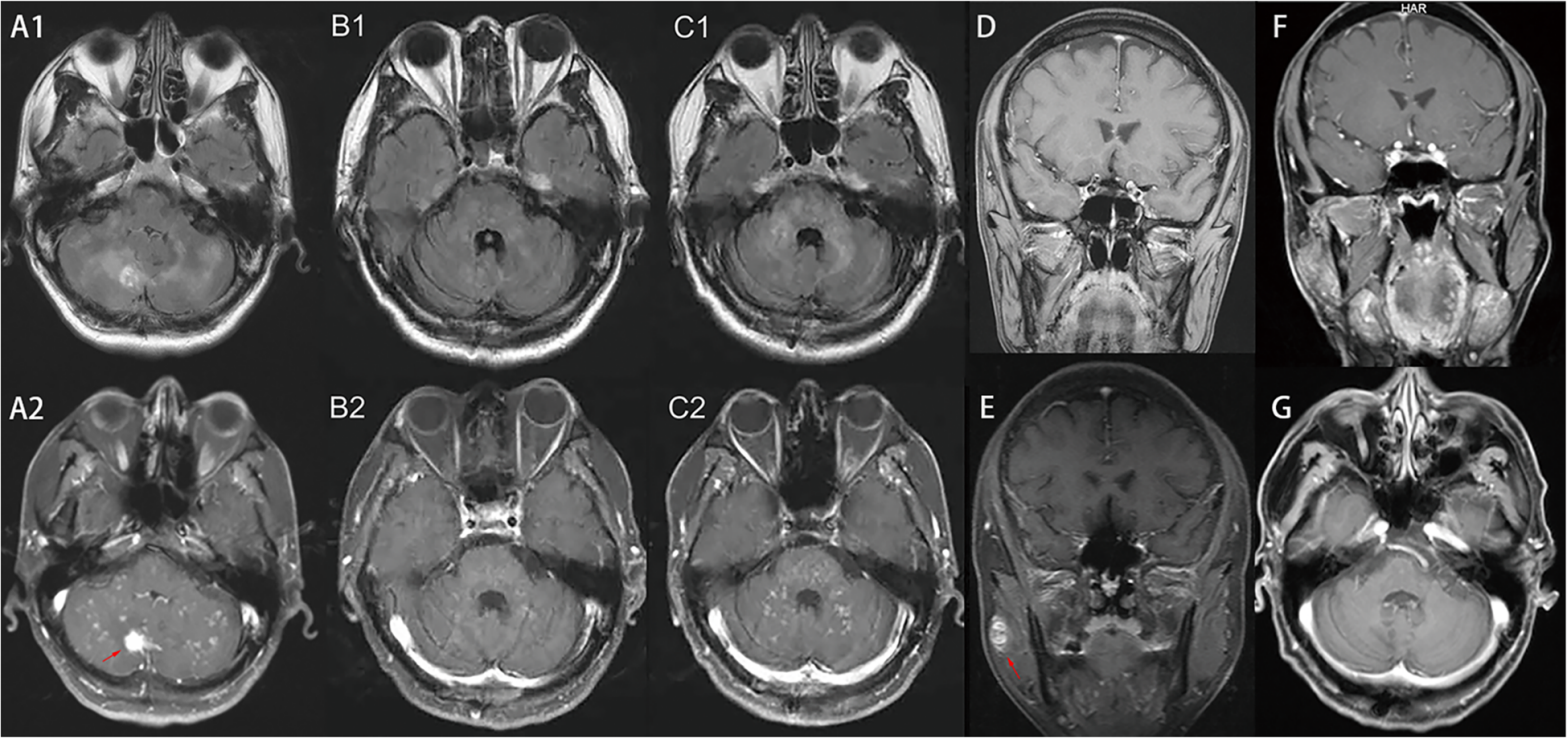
Radiological findings. Flair images (A1) and Axial contrast-enhanced T1-weighted (A2) showed punctate and curvilinear enhancing lesions “peppering” on pons and cerebellum, with a large one in 1.07 cm diameter located in the right cerebellar hemisphere (arrow). There was no parotid malignancy observed at that time (D). With pulse therapy of intravenous methylprednisolone and following oral prednisolone, subsequent MRI showed the lesions decreased both in size and amount (B1, B2). When the dosage of prednisolone was tapered to 20 mg two months later, MRI showed recurring lesions (C1, C2). An enhancing nodule of 1.8 cm diameter was found in right masseter muscle (arrow) (E). After the resection of parotid malignancy (F), with adjuvant radiotherapy, PCGE lesions disappeared on MRI (G)
